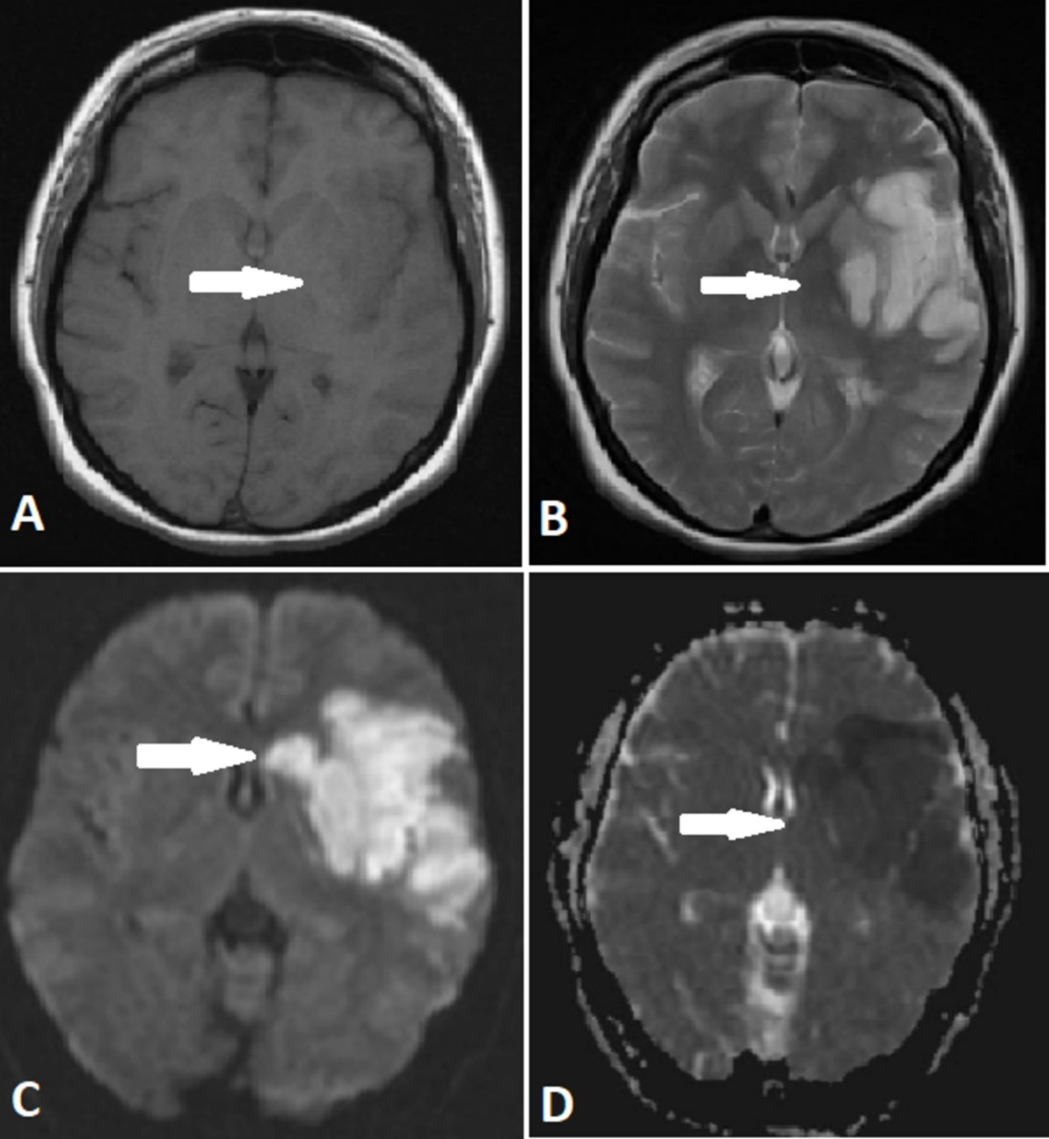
MRI scan of head in axial view (A) T1 scan showing isointense signal in the left parietal region. (B) T2 scan showing abnormal high signals in the left parietal region. (C) Diffusion-weighted 1 (DW1) scan showing a large area of restricted diffusion in the left parietal region. (D) Apparent diffusion coefficient (ADC) scan showing low signals in a large area of the left parietal region
